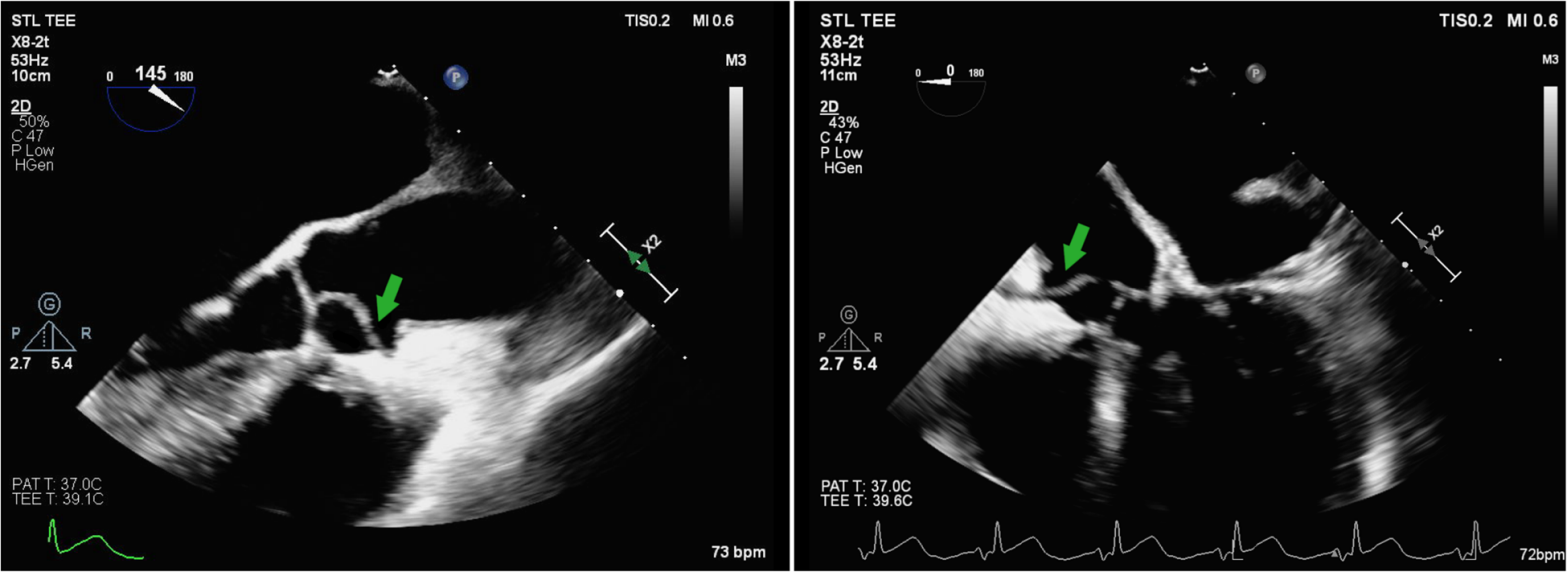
Two transesophageal echocardiography (TEE) views of the aortic valve vegetation (green arrows) showing its prolapse into the right coronary artery ostium
